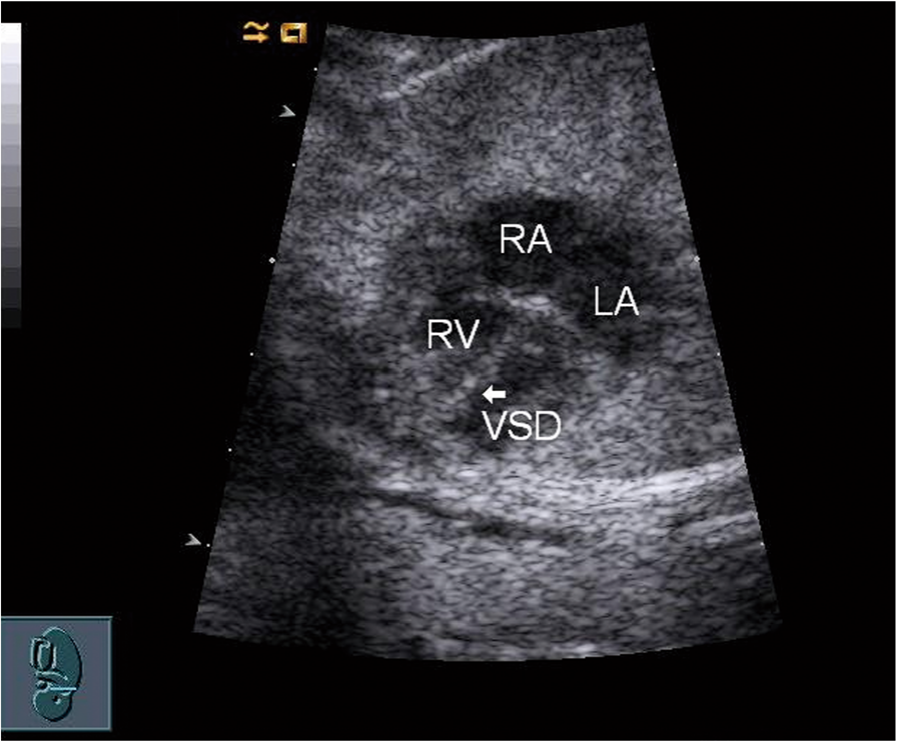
White arrow* indicates a small VSD detected by fetal echocardiography, and SC during follow-up
